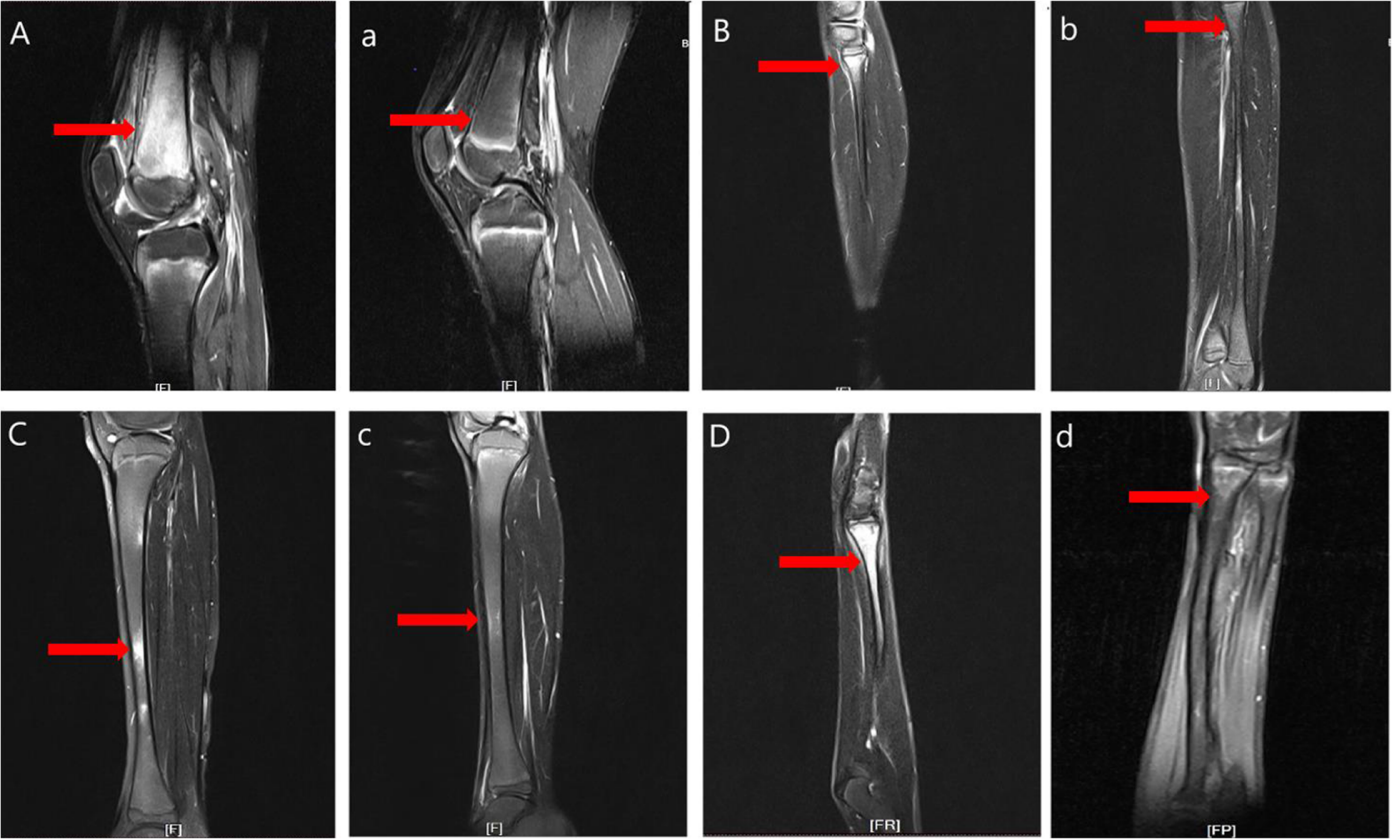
MRI findings in case 13 with chronic nonbacterial osteomyelitis. MRI STIR or T2 show hyperintensity of bone marrow before treatment in metaphysis or diaphysis of femur (A), fibula (B), tibia (C) and radius (D), and remission after treatment (a, b, c, d)
